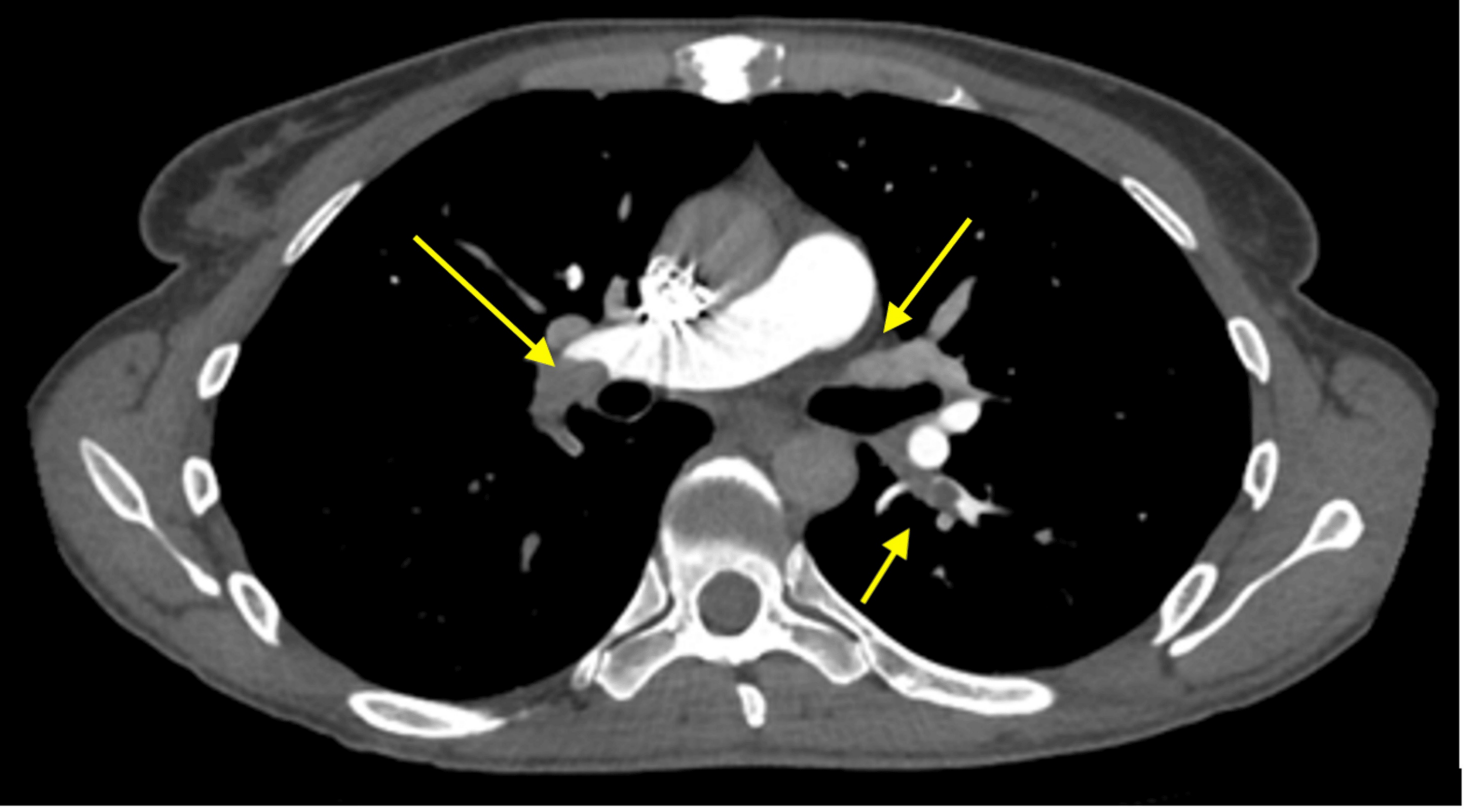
Helical computed tomography pulmonary angiography showing bilateral pulmonary embolism
